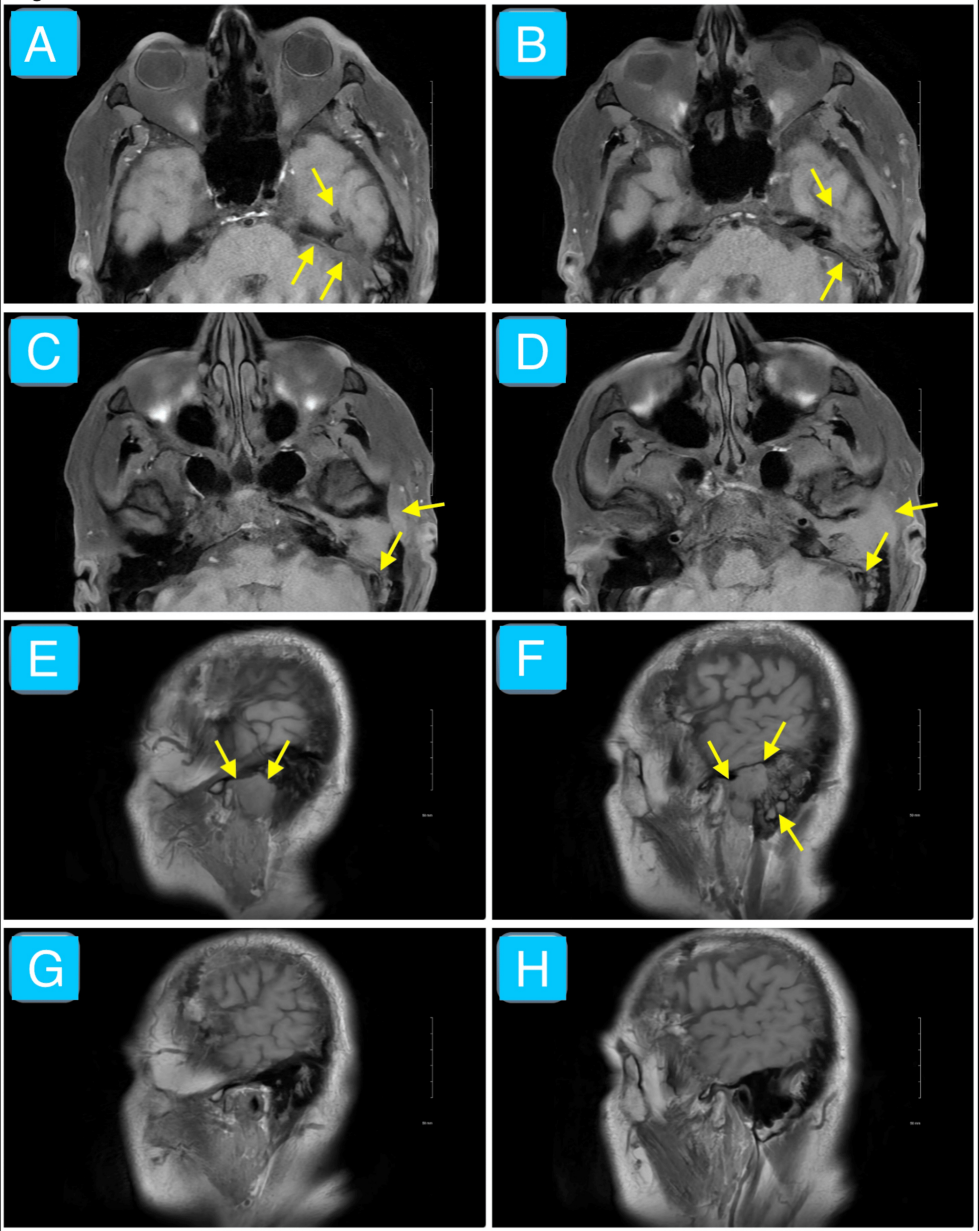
Non-contrast T1-weighted MRI of the face Axial (images A-D) and sagittal (images E-F (left ear), G-H (right ear)) T1-weighted sequence MRI of the face without contrast in a 63-year-old male showing a 3.6 × 1.8 × 3.0 cm poorly defined, mostly homogeneous, hypointense lesion invading the left EAC, middle ear, petrous apex, mastoid bone, posterior TMJ wall, temporal lobe, facial nerve canal, and retromandibular space (yellow arrows). These findings are consistent with an aggressive mass. Biopsy confirmed moderately differentiated SCC. EAC: external auditory canal; TMJ: temporomandibular joint; SCC: squamous cell carcinoma
